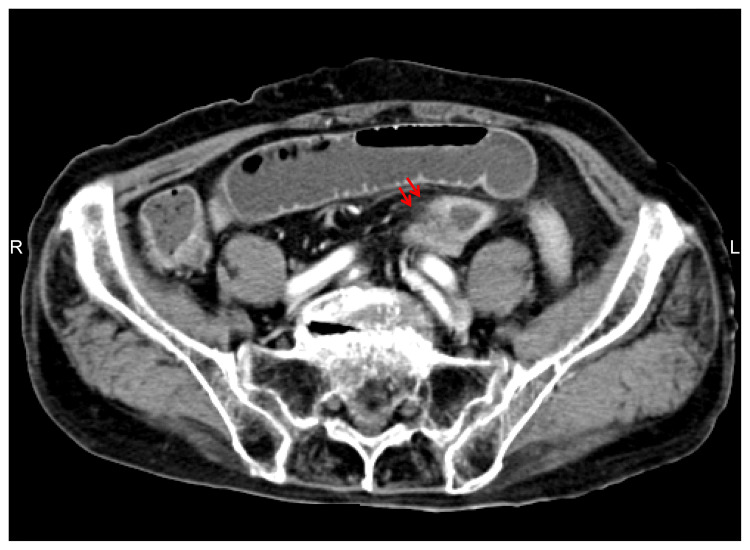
Caliber change (arrow) was revealed on enhanced abdominal computed tomography
